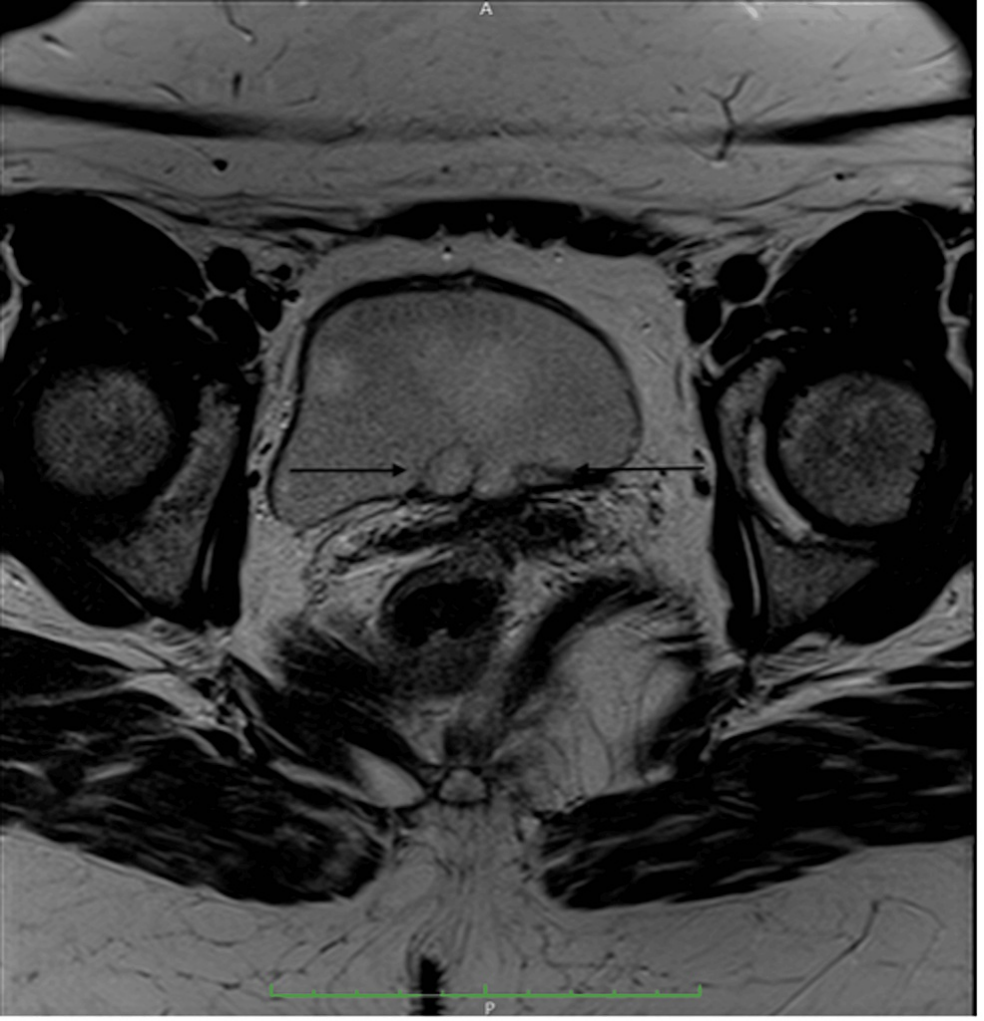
Axial T2 weighted MRI image through the pelvis showing two well-circumscribed structures at the bladder trigone (arrow) which demonstrate a thin rim of low T2 signal with central high T2 signal.
